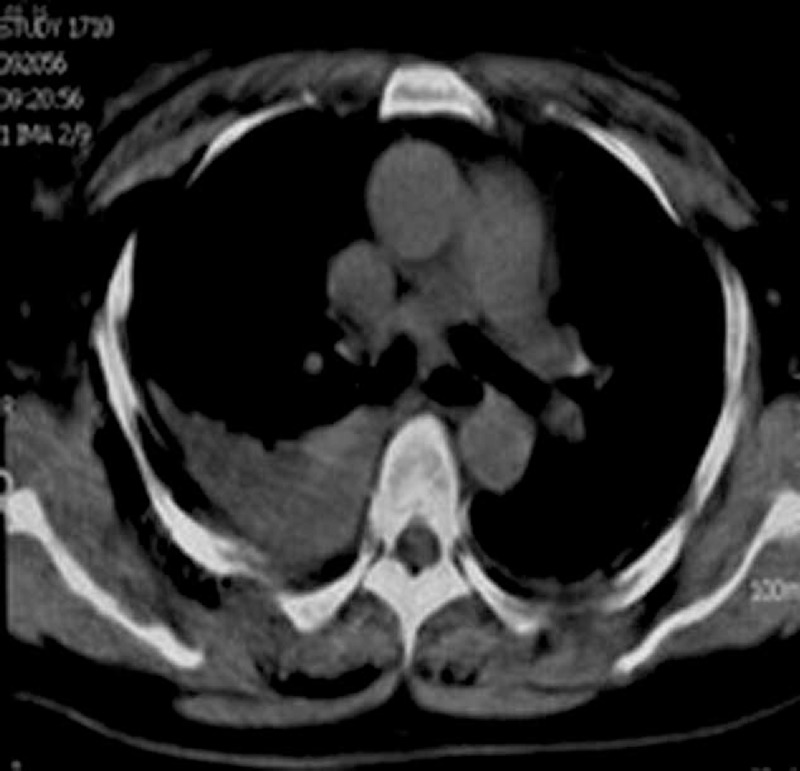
Mediastinal window of chest CT: bilateral patchy shadows, pleural effusion, and hemothorax.
